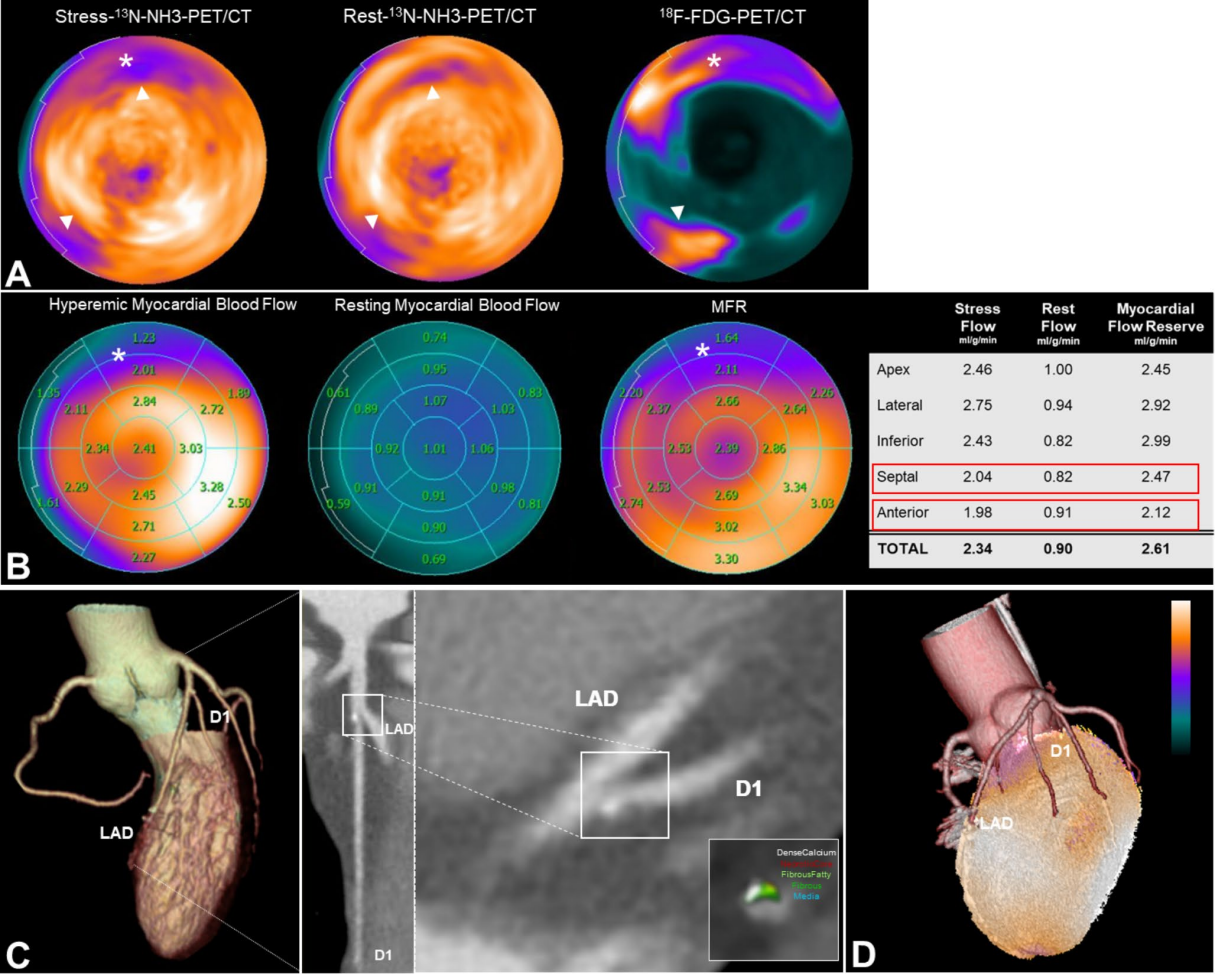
Follow-up Imaging. *A*: ^13^N-NH_3_-PET/CT stress/rest polar plots demonstrating an inferoseptal (fibrosis/scar; arrowhead) and a smaller anterior-basal fixed perfusion defect along with an anterior/anteriorseptal-basal reversible perfusion defect (ischemia; asterisk); and baseline ^18^F-FDG-PET/CT polar plot demonstrating the matched, initially inflamed myocardial regions. *B*: ^13^N-NH_3_-PET/CT myocardial blood flow analysis demonstrating regional (anterior/anteriorseptal) hyperemic myocardial blood flow and MFR reduction, congruent to the ischemic areas (asterisk). *C*: Follow-up CCTA demonstrating discrete progression of the now fibrocalcific ostial D1 lesion, causing borderline obstruction (ca. 50% luminal area stenosis). *D*: Fusion of follow-up CCTA with ^13^N-NH_3_-PET/CT, depicting mismatch of the abnormally perfused areas with the D1 territory
